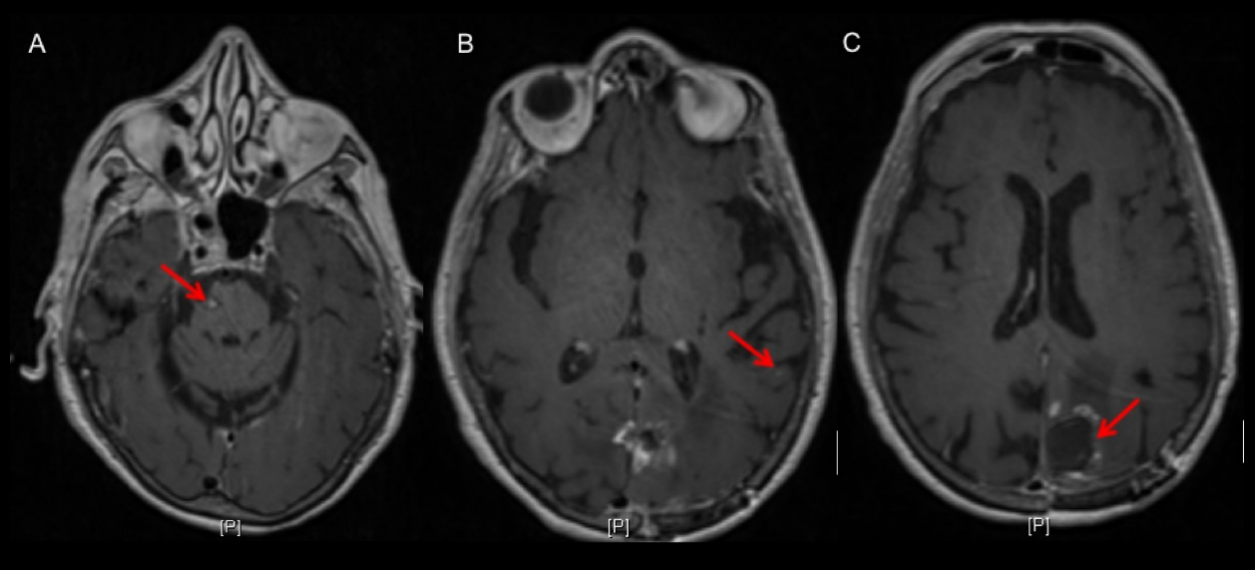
Representative MRI images at patient’s last follow-up Axial images showing stable, small enhancing lesions corresponding to previously treated metastases in the (A) right lateral pons and (B) left supramarginal gyrus. (C) Stable appearance of the left parieto-occipital resection site status post craniotomy and brachytherapy for the resection of a metastasis six years ago and subsequent SRS two years ago for tumor recurrence. SRS: stereotactic radiosurgery
